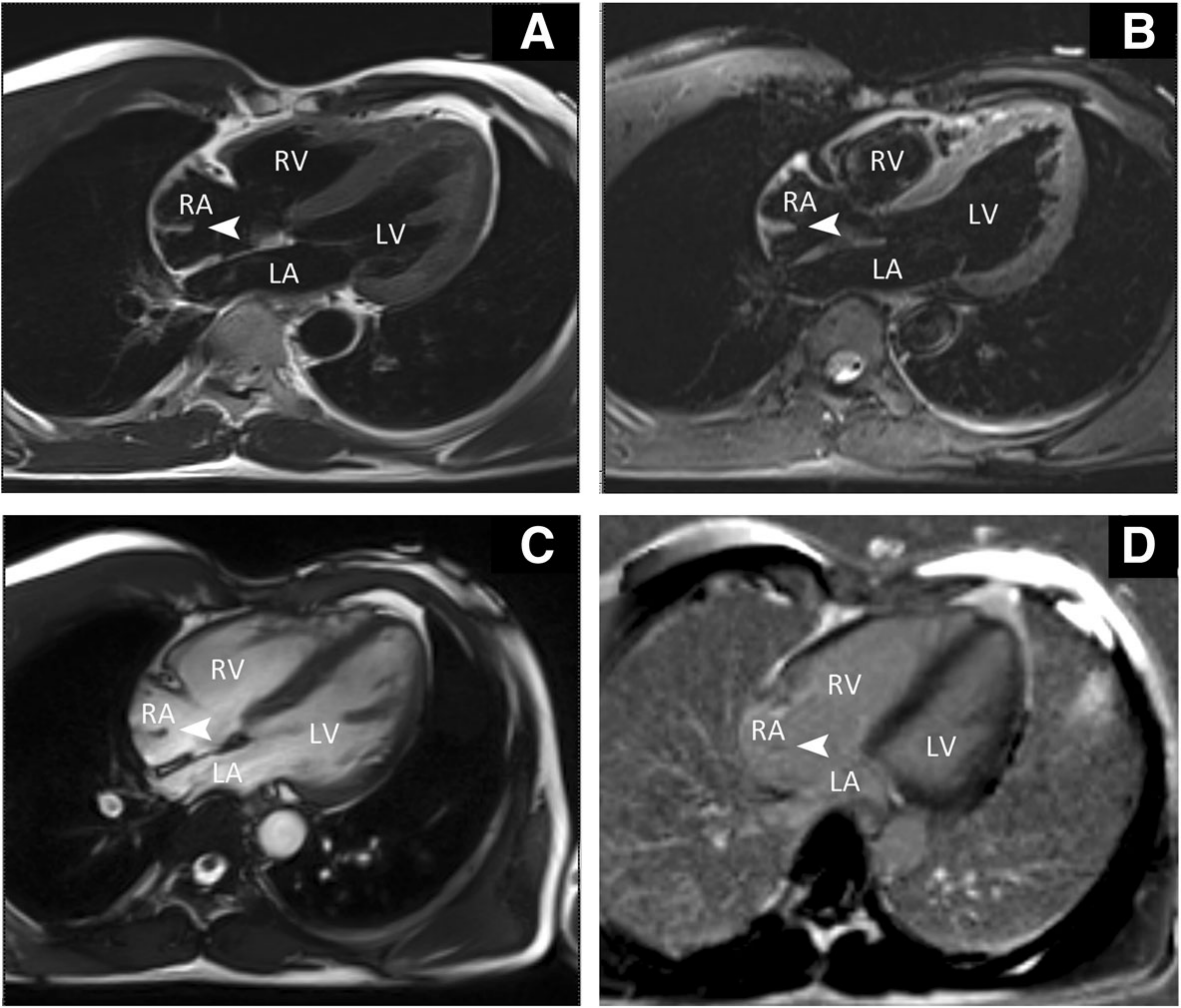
Four chamber view. a. On T2-STIR image, the mass (arrowhead) had similar signal intensity to myocardium revealing a myocardial structure and excluding lipoma. b. On T2-STIR with fat suppression image, the consequence is consistent with (a). c. The cine MRI confirmed a mass (arrow-head) attached to the posterior wall of the right atrium. D. LGE-sequences indicated no abnormal enhancement of the mass (arrow-head). T2-STIR, T2-weighted short tau inversion recovery; LA, left atrium; LV, left ventricle; RA, right atrium; RV, right ventricle; LGE, late gadolinium enhancement
